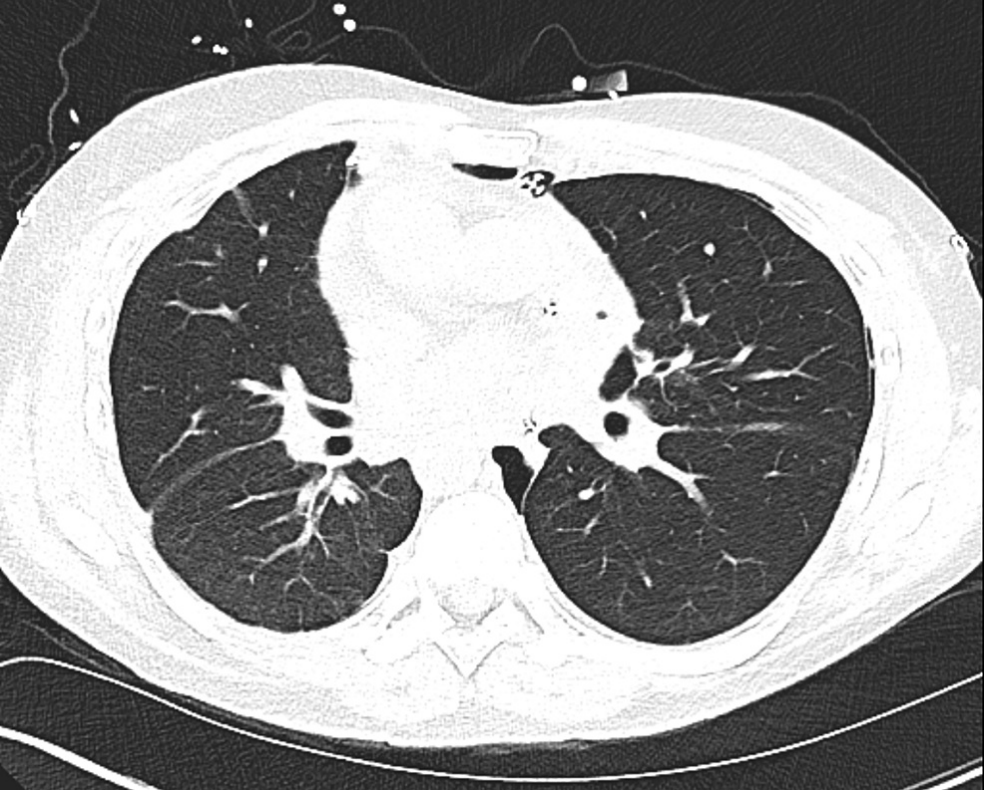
CT chest prior to hospital discharge
